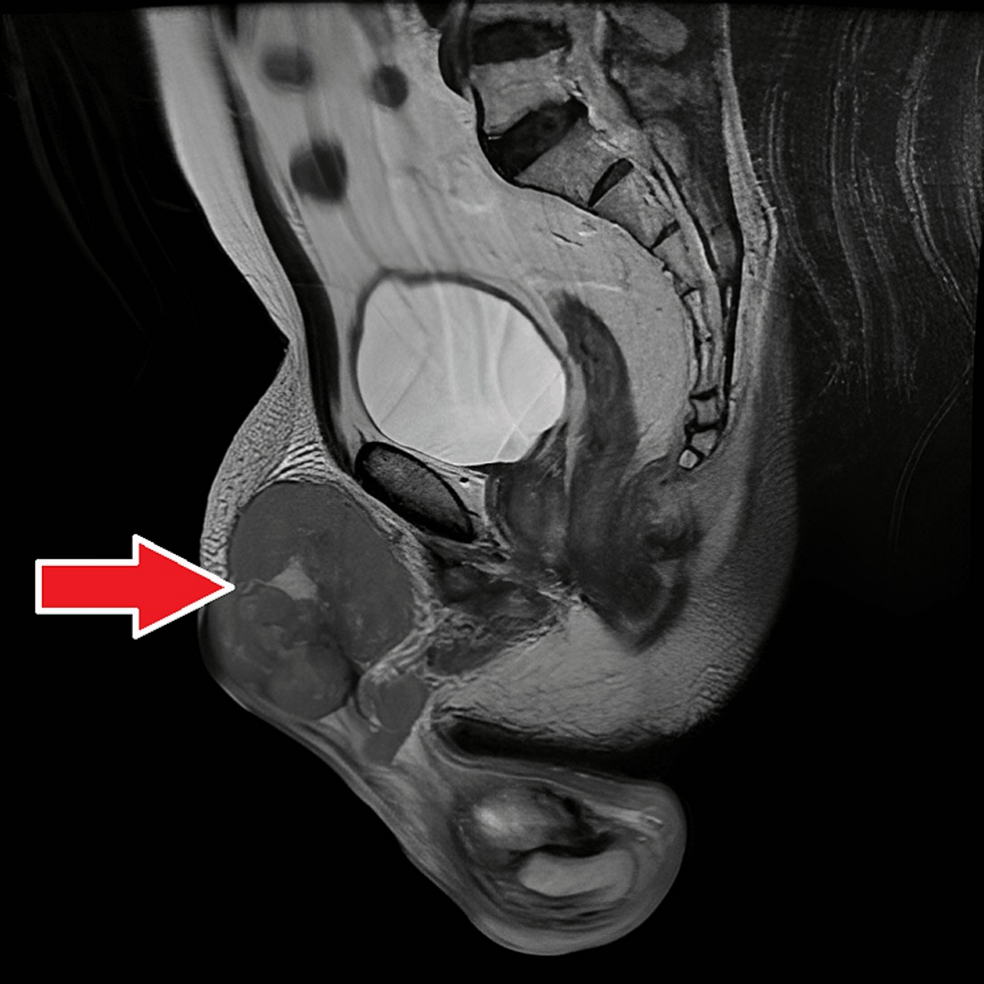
MRI - T2 without fat suppression The image demonstrates sagittal T2 without fat suppression sequence isointense heterogenous mass lesion in the right inguinal region; the mass lesion is interspersed by a few hyperintense areas MRI: magnetic resonance imaging
